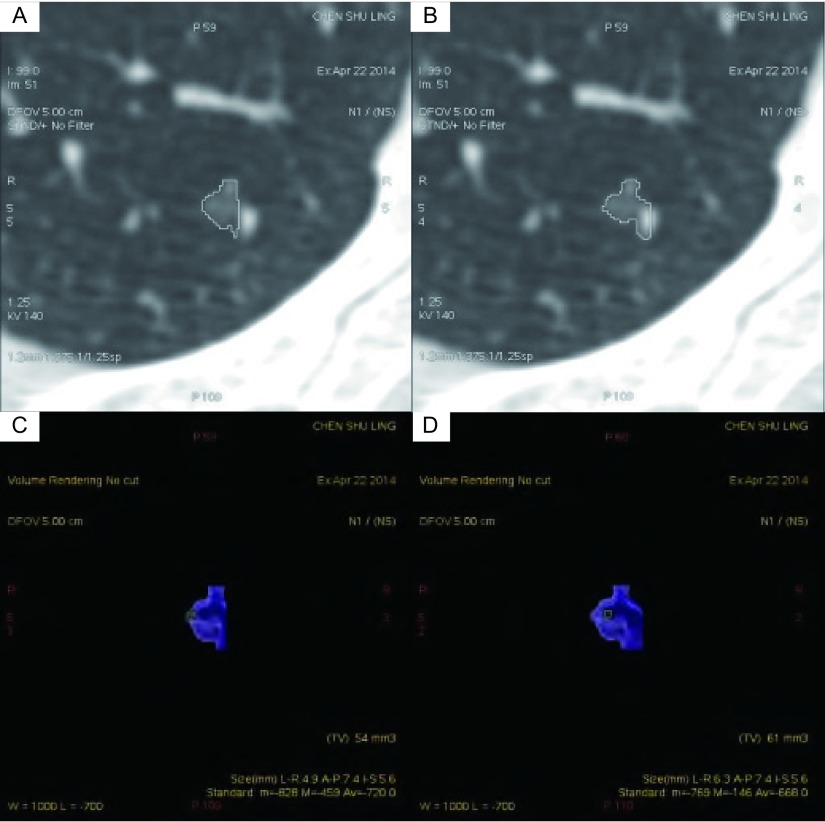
女，55岁，右下叶磨玻璃密度结节，直径约为6.0 mm。图A、C为观察者1第一次测量，大小为54 mm^3^，密度为280 mg/cm^3^，质量约为15.120 mg。图B、D为观察者1第二次测量，大小为61 mm^3^，密度为332 mg/cm^3^，质量约为20.252 mg。质量变异程度与体积变异程度分别为29.0%、12.2% Volume and attenuation measurement of GGN in a 55-year-old woman by using semiautomatic software program. Thin-section chest CT image in lung window setting shows 6-mm GGN in right lower lobe. A, C: the 1^st^ measurement of observer 1, the size of the GGN is 54 mm^3^ and the atteunuation is -720 HU, so the mass is about 15.120 mg; B, D: the 2^nd^ measurement of observer 1, the size of the GGN is 61 mm^3^ and the atteunuation is -668 HU, so the mass is about 20.252 mg. The variation of the mass and the volume is 29.0%, 12.2%. CT: computed tomography
